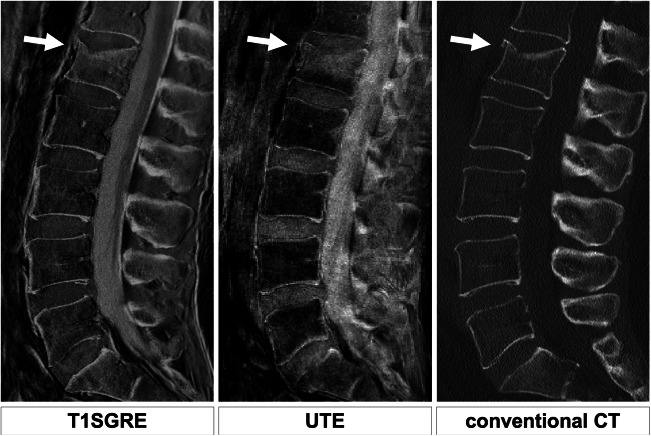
Comparison of T1SGRE-derived CT-like images (left), UTE images (middle), and conventional CT images (right) of the same patient. While the acute compression fracture of L1 (Genant °I, AO/Magerl A1) can be identified and classified in all modalities; the T1SGRE sequence shows a more homogeneous signal and tissue contrast optimal for the assessment of osseous structures. Of note, due to the inverted-grayscale T1w contrast, ligaments are also shown as hyperintense which must not be misinterpreted as calcifications
